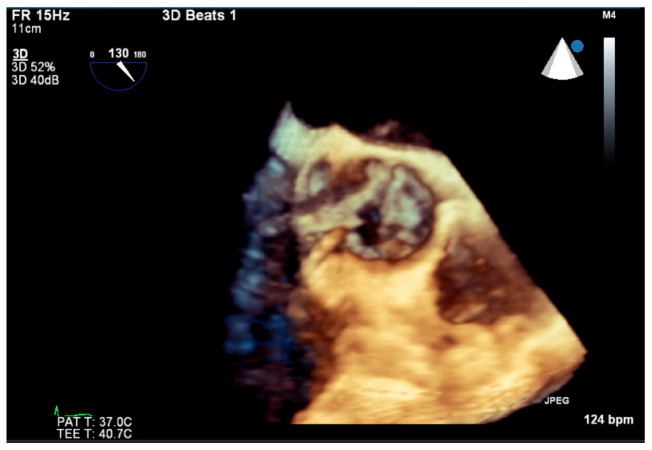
Three-dimensional reconstruction of transesophageal echocardiogram mid-esophageal aortic valve short-axis view demonstrating aortic valve destruction with a large hole in the center.
